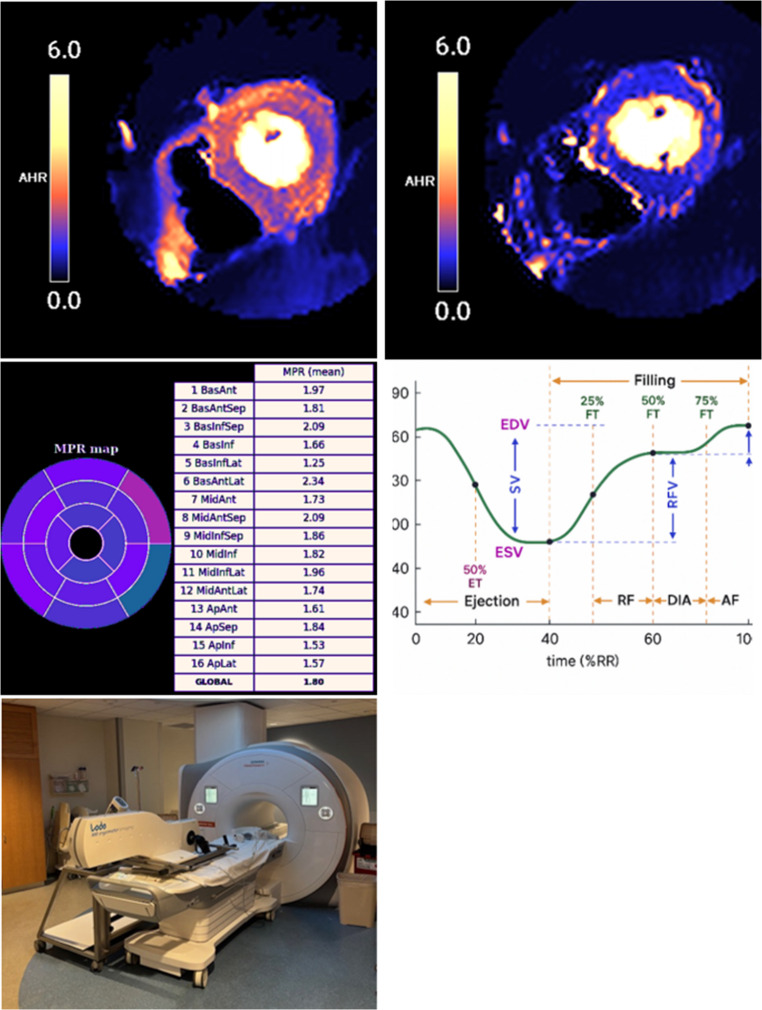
Top left: color map showing rest perfusion sequence with normal perfusion. Top right: color map showing globally reduced stress myocardial blood flows in a HFpEF patient. Middle left: globally reduced MPR of 1.8 consistent with CMD. Middle right: time volume curve tracking ventricular filling throughout the cardiac cycle. (From Goyal N et al. [39], with permission from Elsevier.) Bottom: MRI compatible exercise bike for stress CMR. CMD – coronary microvascular disease. HFpEF – heart failure with preserved ejection fraction. MPR – myocardial perfusion reserve. CMR – cardiac magnetic resonance
